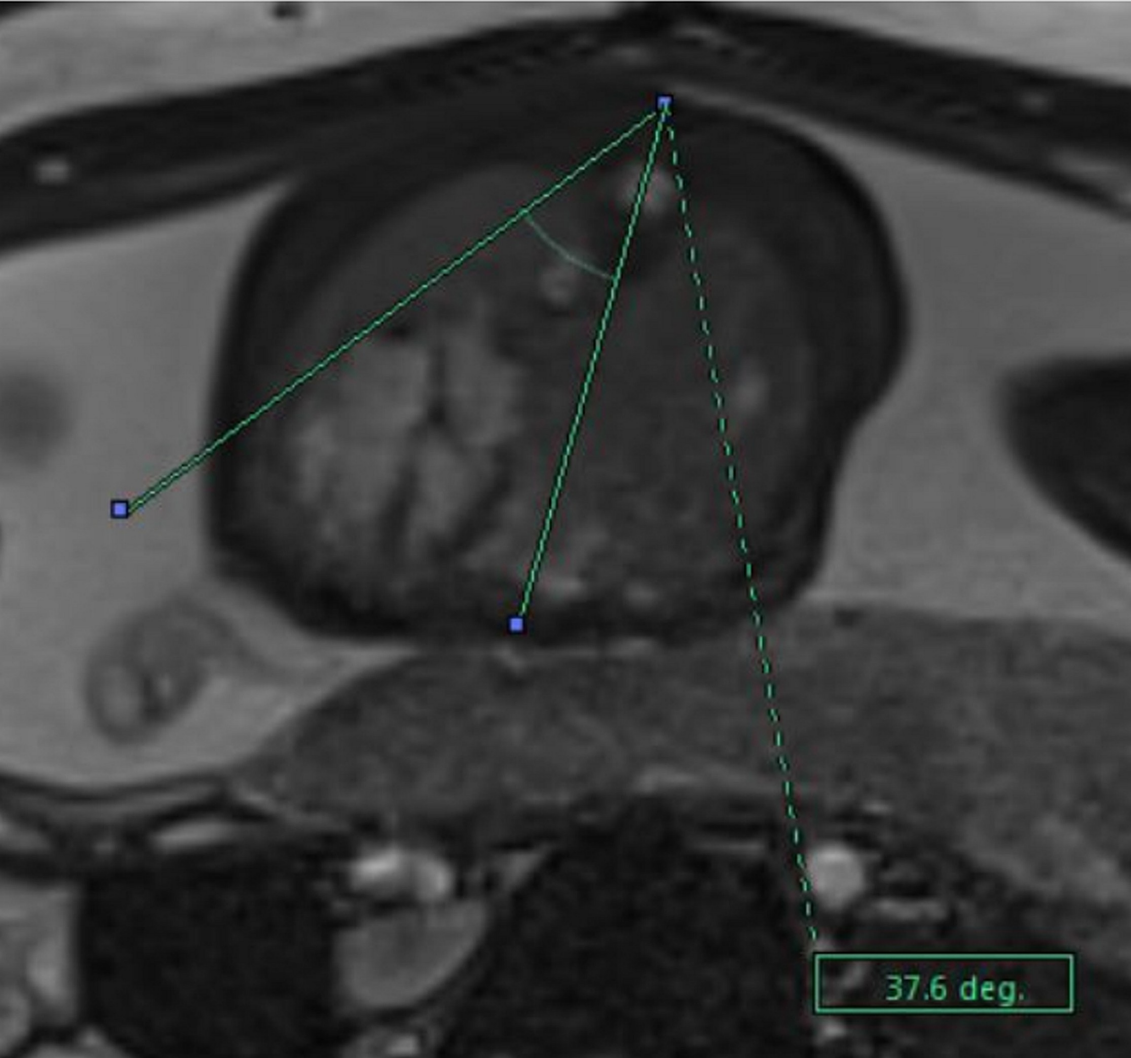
Mediastinal shift angle. MRI calculation of the mediastinal shift angle (MSA) on axial TrueFisp-sequences.
